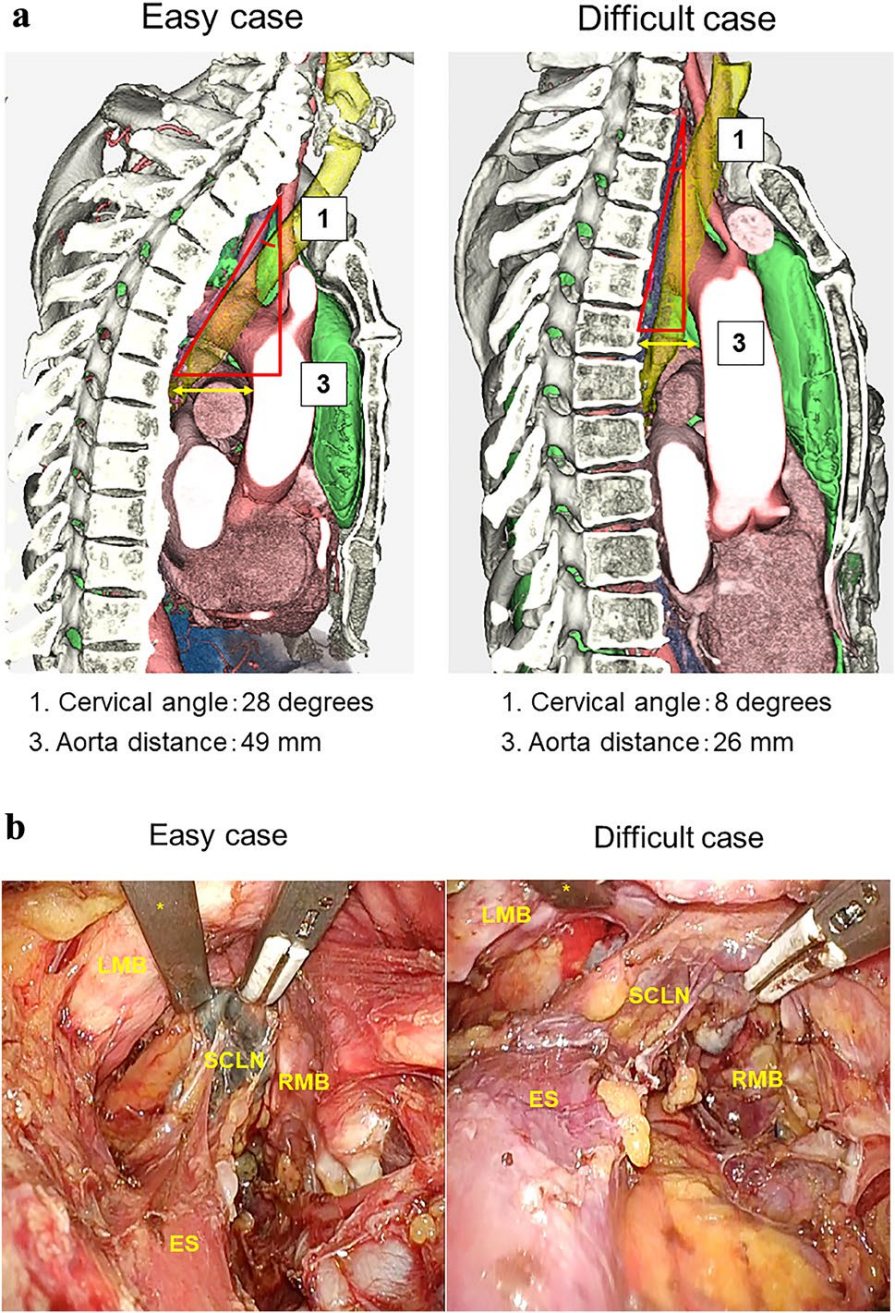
Representative images according to the difficulty of SCLN dissection. Preoperative 3D-CT image (A) and surgical view (B) in easy and difficult cases are shown. In the surgical view, SCLN (subcarinal and right main bronchial lymph nodes) stood up in a wide space between the tracheal carina and esophagus in an easy case, while in a difficult case, SCLN appeared to lie down in the narrow space. ES, esophagus; LMB, left main bronchus; RMB, right main bronchus; asterisk, left-hand retractor
